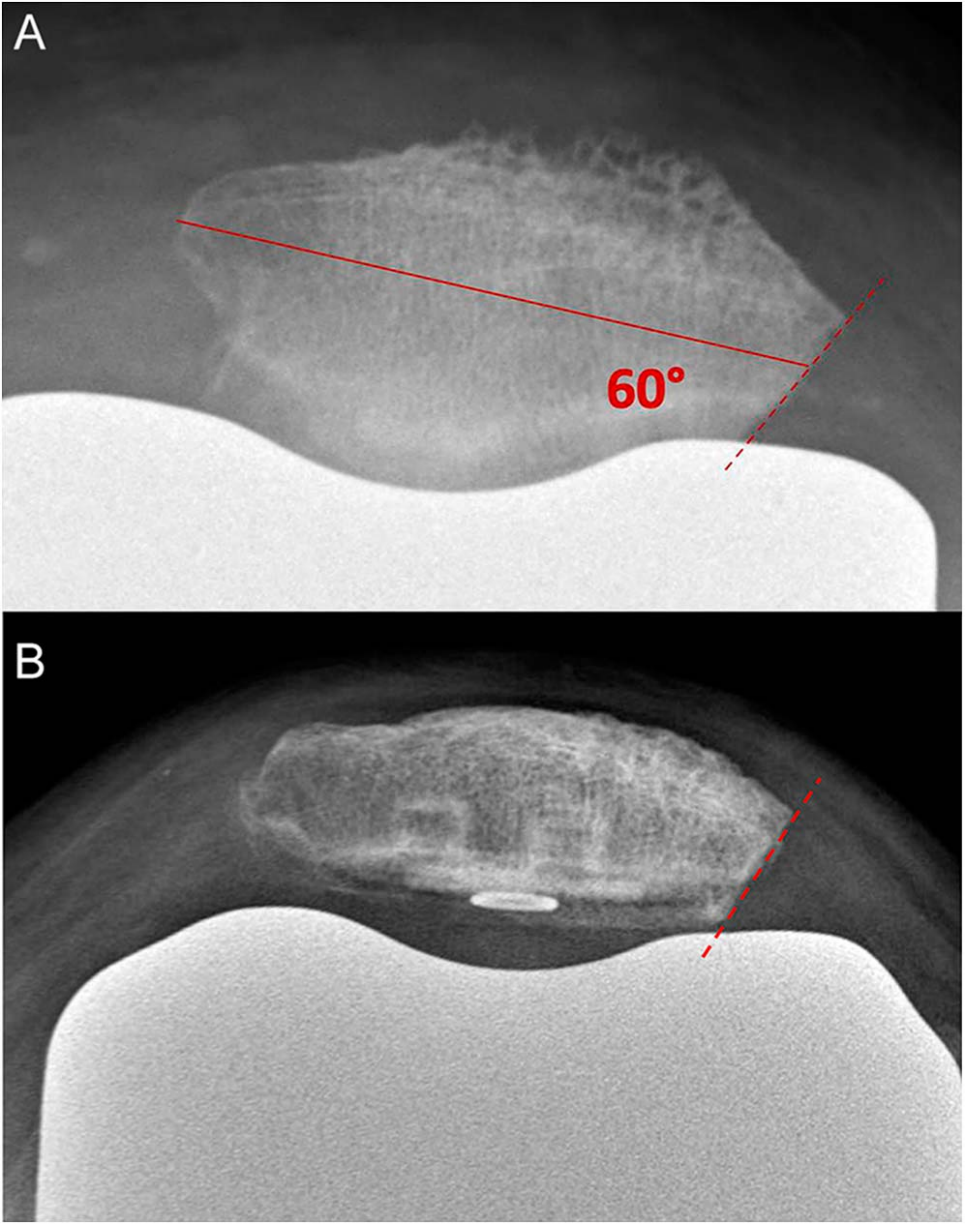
Postoperative radiographs after partial lateral facetectomy (skyline view). (A) Un-resurfaced patella; (B) resurfaced patella.
